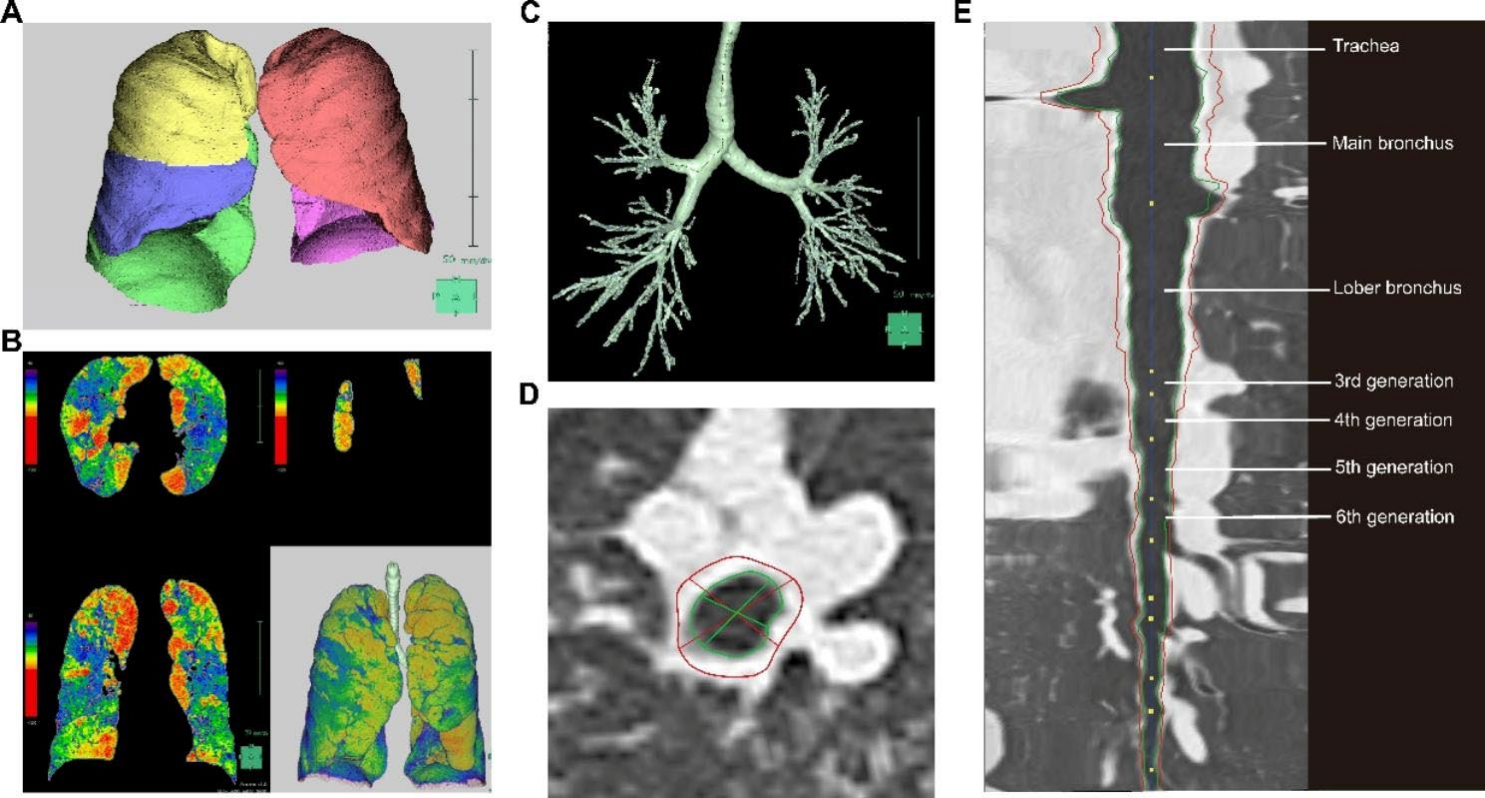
3D analysis of chest CT using SYNAPSE VINCENT. (A) Lung lobe extraction and segmentation. (B) Low attenuation area (LAA%) distribution analysis, the closer to red means more severe emphysema, the closer to blue means less severe emphysema. (C) 3D CT reconstruction image of the airway tree. (D) Cross-sectional images of selected airways, with the red line indicating the outer wall and outer diameter of the airway and the green line indicating the inner wall and inner diameter of the airway. (E) Longitudinal section image of the selected airway, yellow dots are automatically marked branch points
